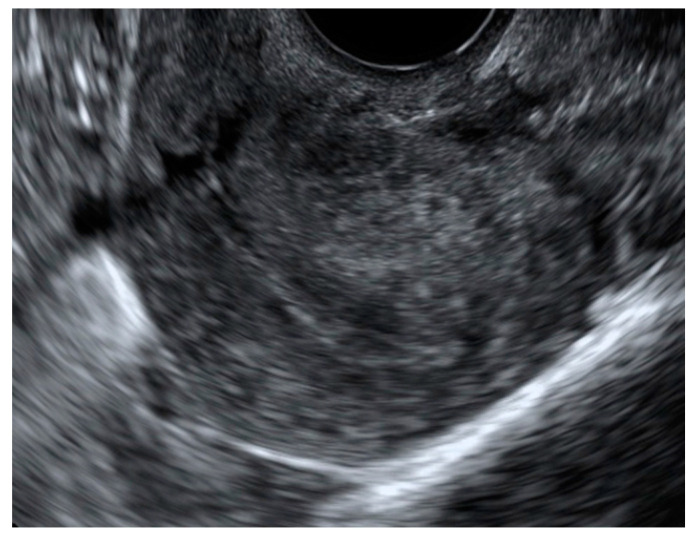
Transvaginal two-dimensional ultrasound images of a uterus affected by diffuse adenomyosis of the external myometrium and deep infiltrated endometriosis (DIE).
